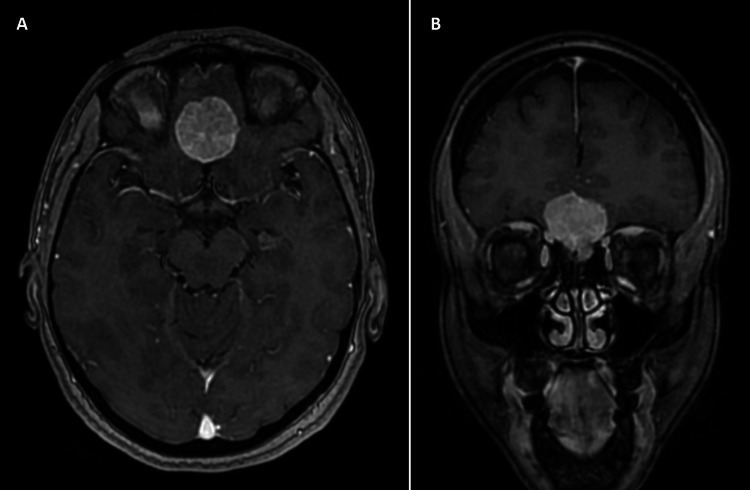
Preoperative MRI Preoperative gadolinium-enhanced T1-weighted images in axial (A) and coronal (B) views showing a well-demarcated and homogenously enhancing mass lesion situated at the midline of the anterior fossa of the skull base.
